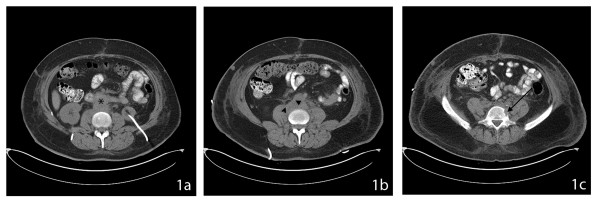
CT Scans of the Abdomen. 1a:. CT scan demonstrates the presence of a soft tissue mass (*) impinging on the duodenum. 1b: CT scan demonstrates the presence of an extensive, abnormal retroperitoneal soft-tissue mass (▲) surrounding the aorta most consistent with metastatic lympadenopathy. 1c: CT scan also shows evidence of some lytic destruction of the left lateral aspect of the L5 vertebral body most likely related to local invasion of the metastatic disease.
